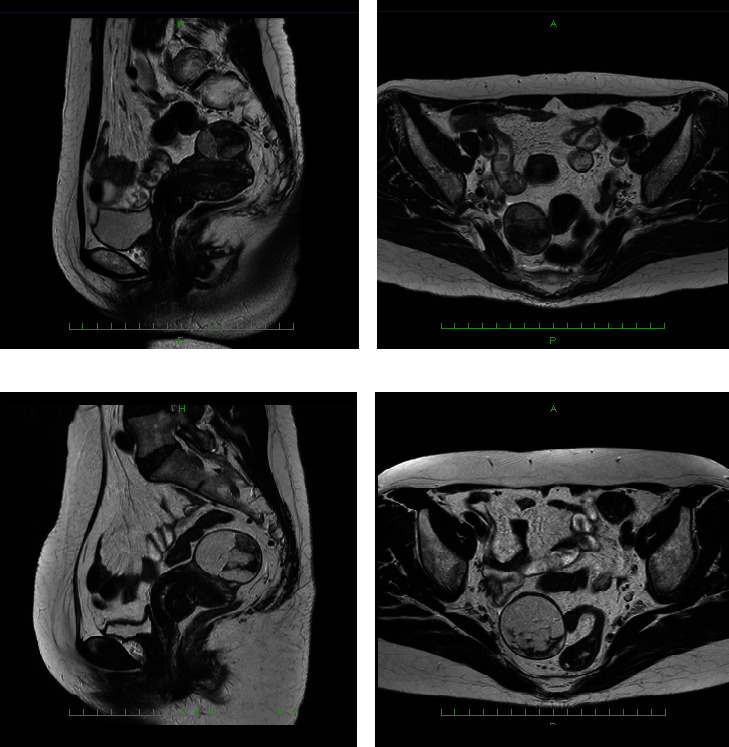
Preoperative MRI at 52 and 58 years old: (a) T2-weighted image at 52 years old presenting the maximum section of the mass in sagittal plane; (b) T2-weighted image presenting the maximum section of the mass in the transverse plane; (c) T2-weighted image at 58 years old presenting the maximum section of the mass in the sagittal plane; (d) T2-weighted image presenting the maximum section of the mass in the transverse plane. The average diameters of the major and minor axes on the sagittal plane and the major diameter of the transverse plane increased by 1.23-fold. The tumor volume increased from 27 to 49 mL (1.85-fold) when approximated by the elliptical sphere formula.
